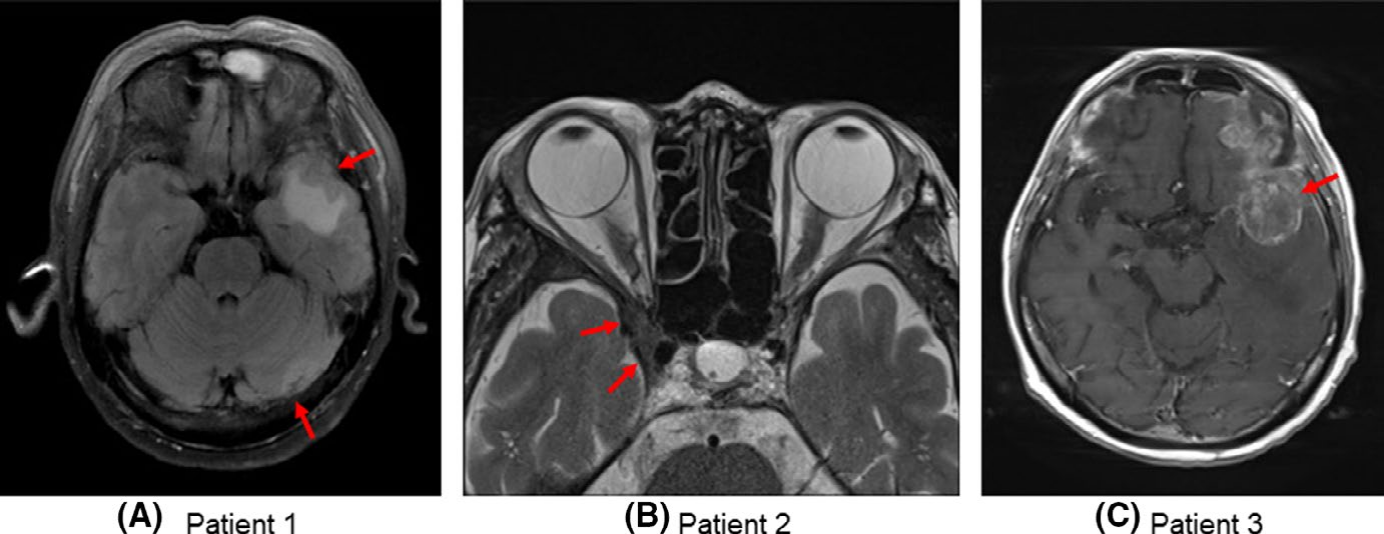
Cranial MRI imaging of three patients. Arrows show metastatic sites. A, Patient 1: Plain cranial MRI showed left middle cranial fossa metastasis. B, Patient 2: Plain cranial MRI showed right cavernous sinus metastasis. C, Patient 3: Gadolinium (Gd) contrast‐enhanced cranial MRI showed a brain metastasis of 3.5 cm in diameter in the left middle cranial fossa
